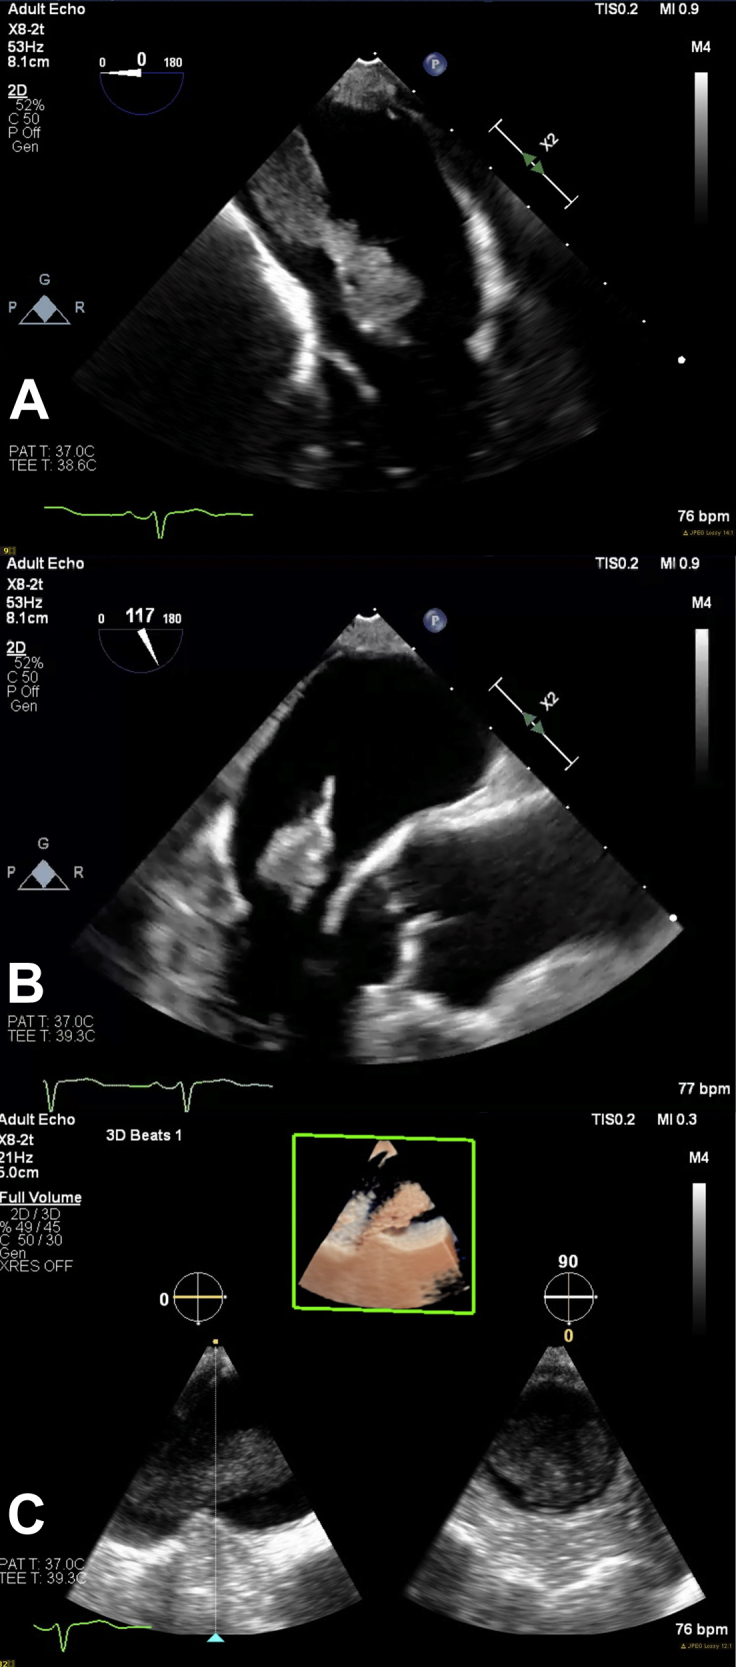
Transesophageal echocardiogram demonstrating a LA mass originating from the right lower pulmonary vein. The mass has a fixed component (39.0 × 13.3 mm) and a friable mobile segment (12.4 × 8.5 mm). (A) Four-chamber 0° zoom view of the LA mass. (B) Three-chamber zoom view at 117°. (C) X-plane of the mass originating from the right lower pulmonary vein.
